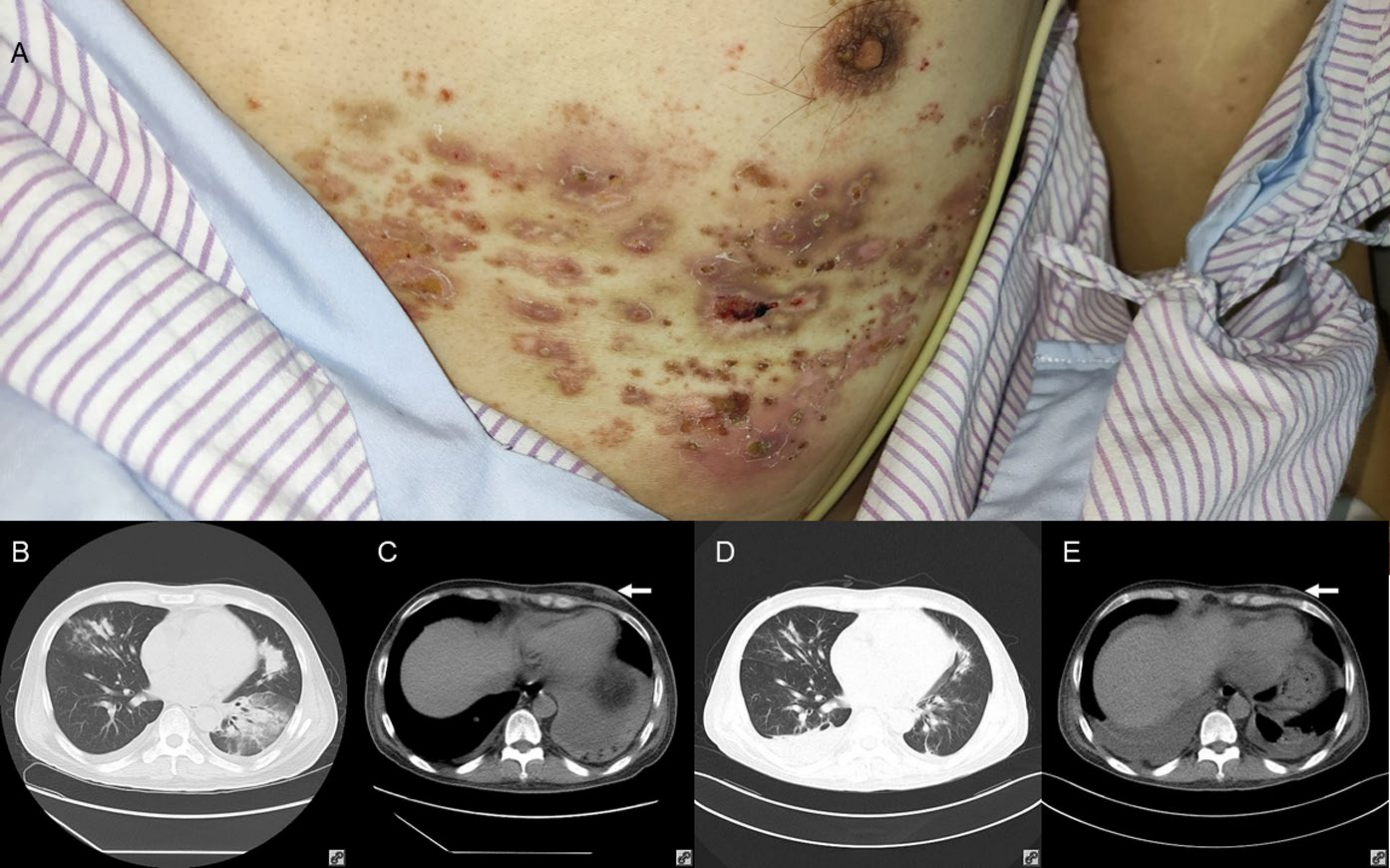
Clinical and radiological assessment of the index patient. Panel A: Clinical photo on admission, showing subcutaneous nodules on left chest wall and crusting due to healing herpes zoster. Panel B: Thoracic computerized tomography (CT) on admission, showing multiple patchy infiltrates and consolidation with air bronchograms in both lungs and bilateral pleural effusion. Panel C: Mediastinum window of thoracic CT on admission, showing multiple subcutaneous nodules of low density in the left chest wall (arrow). Panel D: Thoracic CT two weeks after commencement of antifungal therapy, showing resolving lung infiltrates and consolidation, and residual pleural effusion. Panel E: Mediastinum window of thoracic CT two weeks after commencement of antifungal therapy, showing shrinking of the subcutaneous nodules in left chest wall (arrow)
